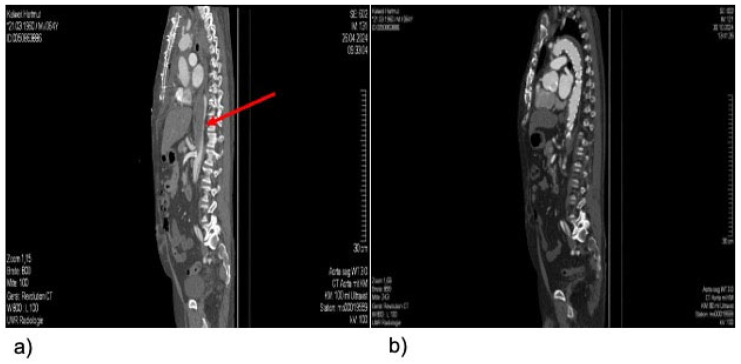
(a) Sagittal view from a CT examination of an exemplary patient with clinical signs of malperfusion following proximal aortic repair (FET), due to a nearly collapsed true lumen in the descending thoracic aorta (indicated by red arrow). (b) Sagittal view from a CT-examination of an exemplary patient (same patient as in Figure 1), demonstrating the postoperative result in the descending thoracic aorta following urgent TEVAR.
